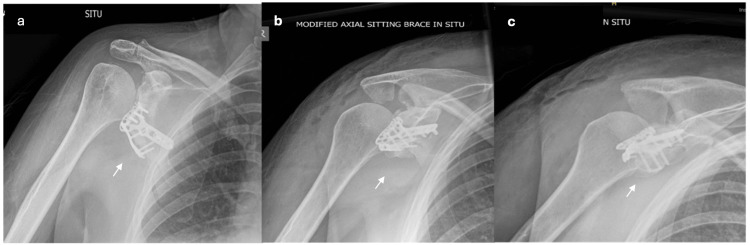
Day one post-operative radiograph of the right shoulder. a. Anterior-posterior view, b. axial view, and c. modified axial view. Figures 3a-3c display the maintained reduction and position of the distal radius plate at post-operative day one (as indicated by arrows).
